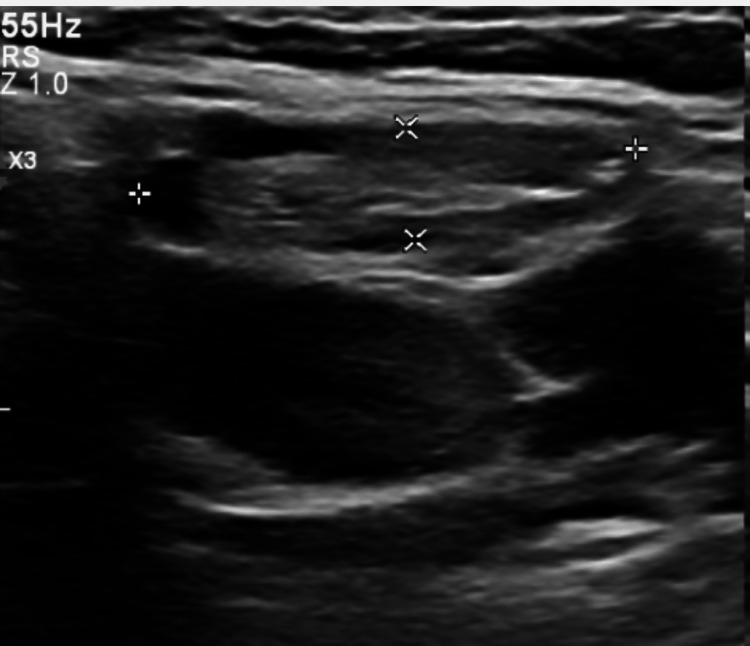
Ultrasound of the right parotid gland.
